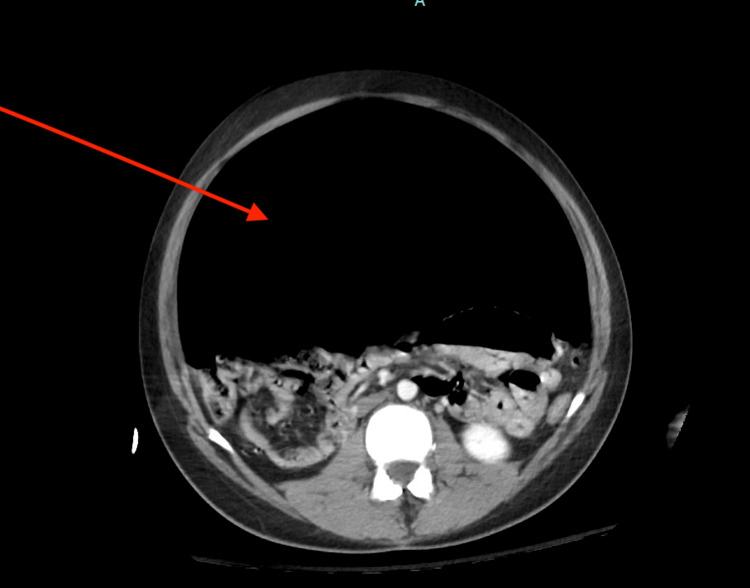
Axial View CT scan of the Patient With Intravenous Contrast Prior to Laparotomy Indicating Significant Free Air in the Abdominal Cavity, Highlighted by Red Arrow
